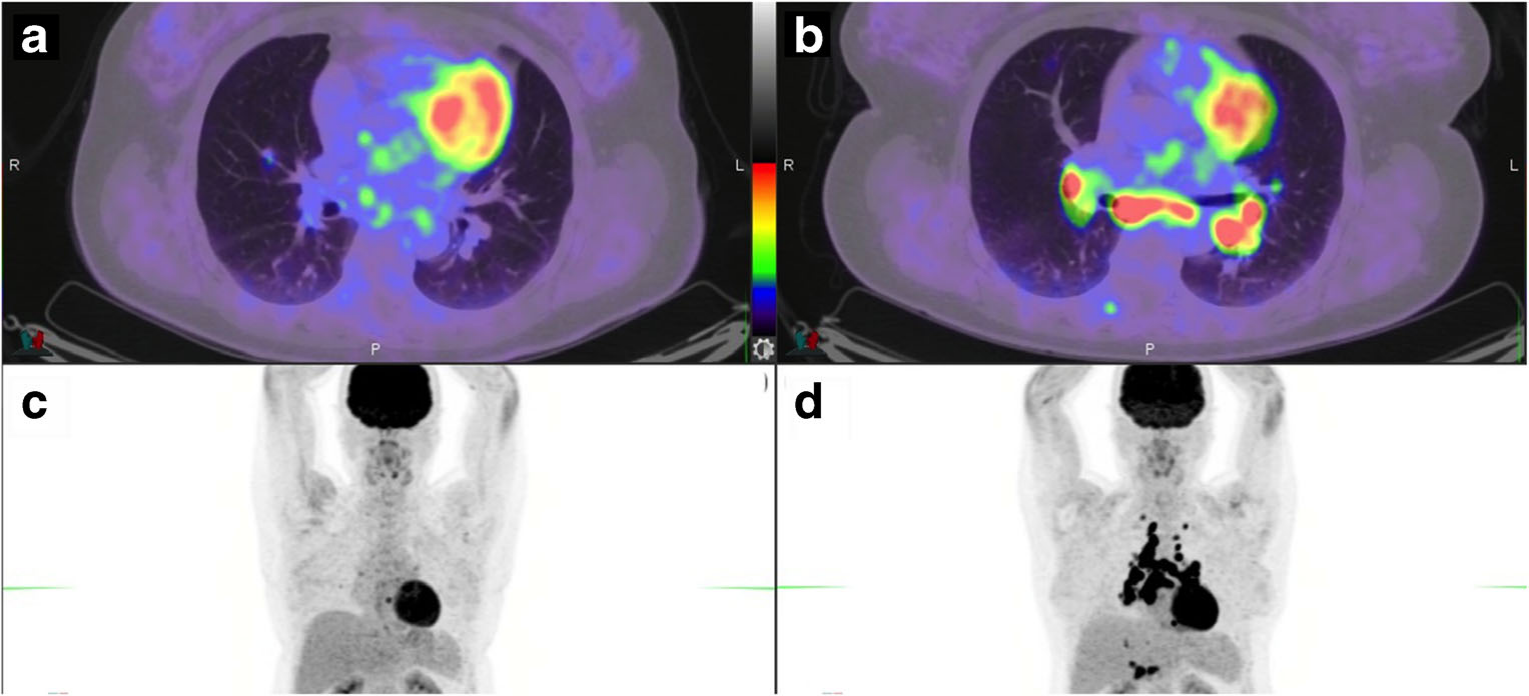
A patient with new pulmonary metastases (a fused PET/CT image, c maximum intensity projection image). Following treatment with pembrolizumab (b fused PET/CT image, d maximum intensity projection image), dramatic uptake is seen in symmetrical hilar, mediastinal and portocaval nodes indicating treatment-induced sarcoidosis. Prior small pulmonary nodules have resolved
